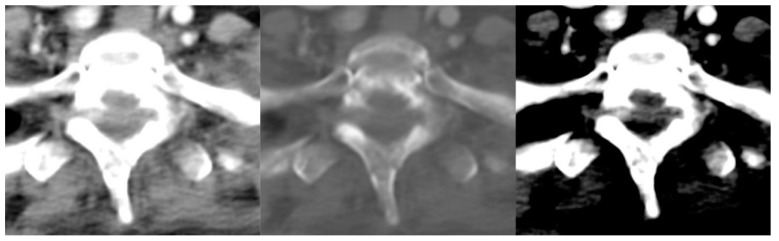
Axial CT image of the cervicothoracic junction overcalled as low-grade epidural disease by two radiologists. No epidural disease was present on the corresponding MRI (not shown). Assessment is complex due to overlap and angulation of the lower cervical and upper thoracic spine in the axial plane with background degenerative changes.
